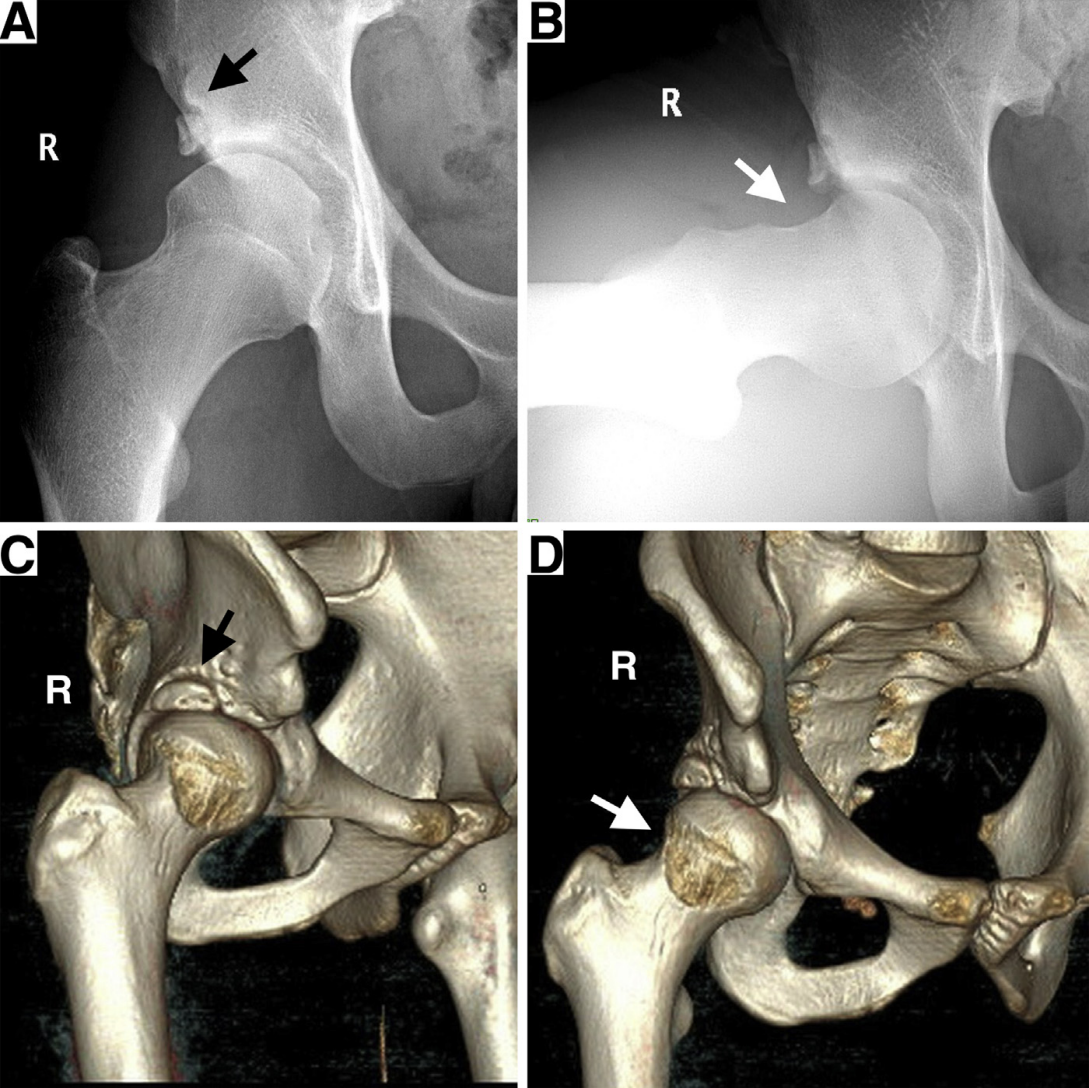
The postoperative anteroposterior view (A) and Dunn view (B) of the right hip plain radiography showing the os acetabuli was well secured (black arrow) and sufficient correction of cam lesion was made (white arrow). The postoperative 3-dimensional computed tomography image with different rotating views (C and D) showing proper fixation of os acetabuli (black arrow) and the complete correction of cam lesion (white arrow).
